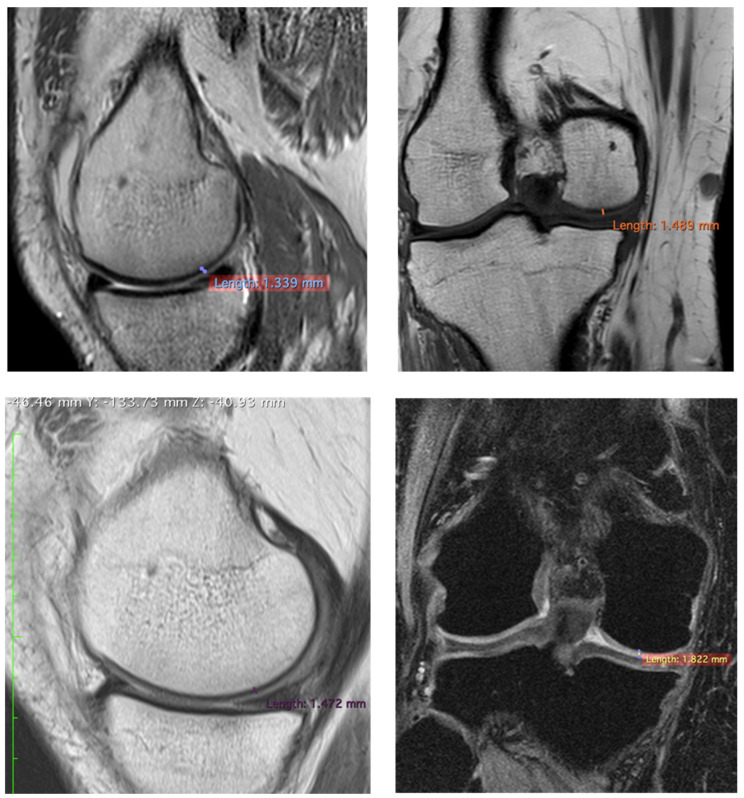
Representative images of MRI of patient no 6 at 3 years. Images of femoral lateral condyles and measurement of thickness cartilage. We state that different MRI machines and different sequences were used for each patient.
